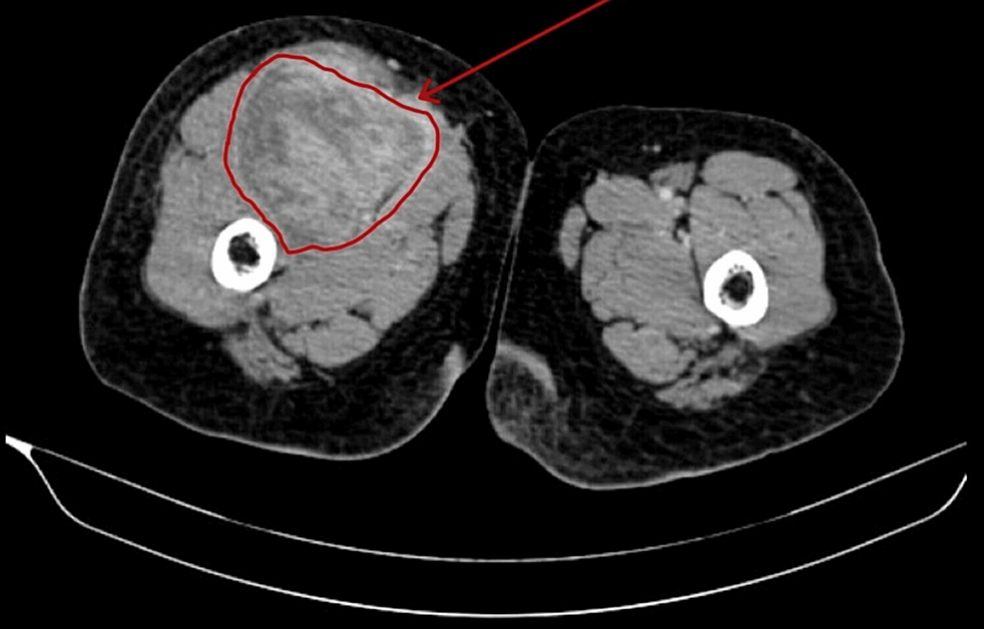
Contrast-enhanced CT thigh venous phase showing a large, well-defined lobulated lesion of size 12.5 x 8 x 8 cm in the intermuscular plane of the anterior aspect of the right upper thigh, along with compression of adjacent muscles.
